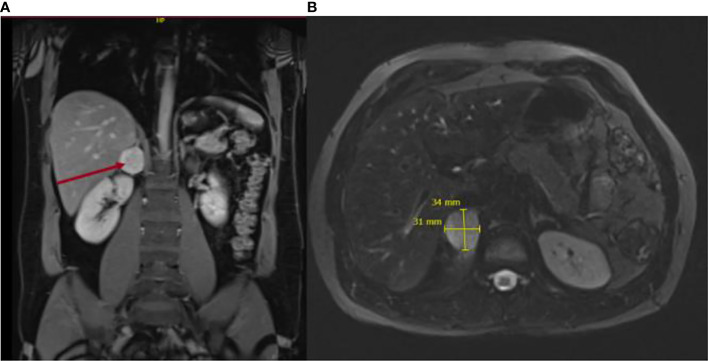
Magnetic resonance imaging depicting axial diffusion-weighted (A) and coronal views (B) of the 4-cm adrenal mass. .
